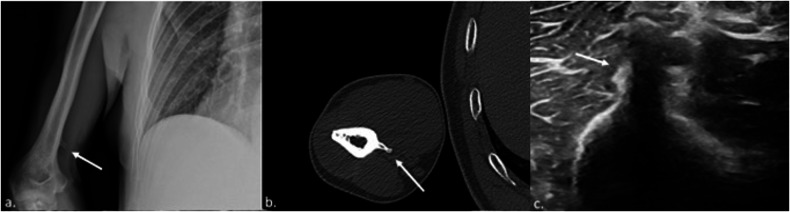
X-ray (a), CT (b), and US (c) of the supracondylar process (arrow)
